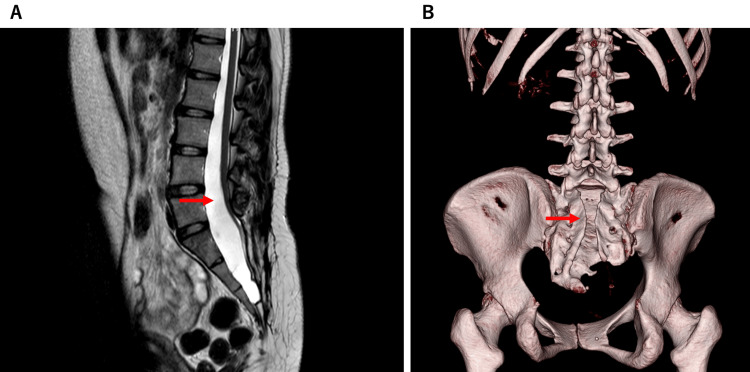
Lumbar and sacral images A. MRI shows a tethered spinal cord. The red arrow points to the low conus medullaris at the L5 level. B. Reconstructed CT images reveal spina bifida in the sacral region.
